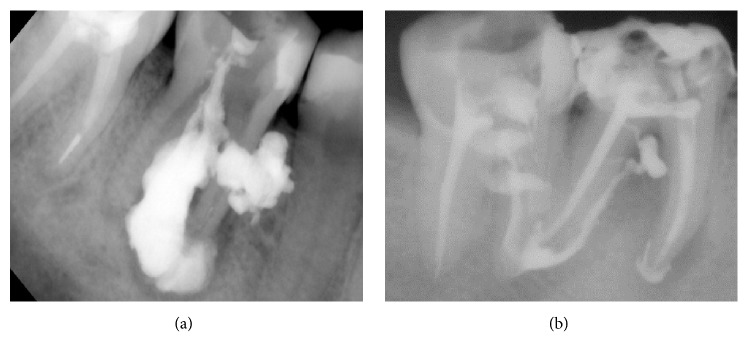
CHISP management can remedy failed root canal treatment of periapical lesion. Recurrence of lesion after failed conventional root canal treatment is high. CHISP offers a nonsurgical treatment with high success rate. (a) Radiograph showing lesions filled with the CHISP through the prepared root canal and buccal-palatal approach. (b) CHISP can be used for smaller lesions.
